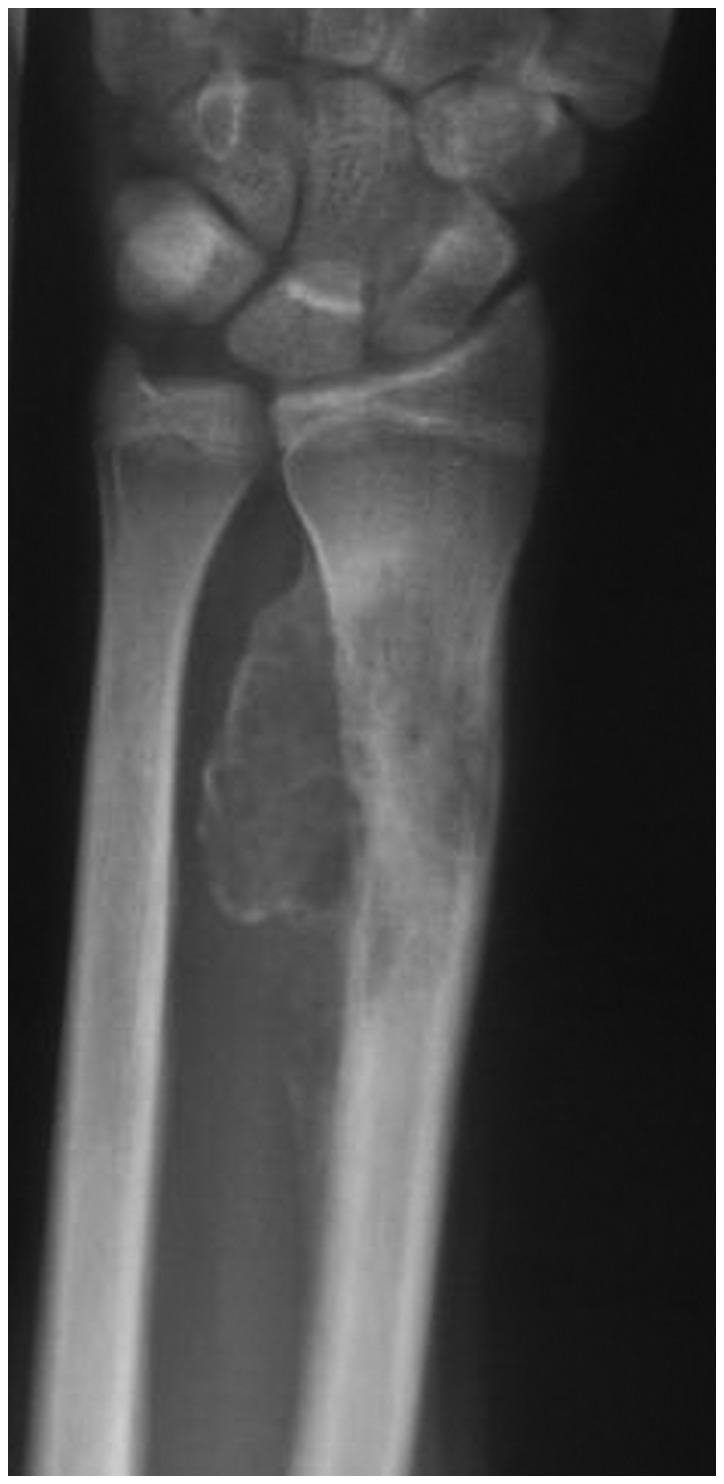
Plain radiography revealed sclerotic changes and a good lesion margin.
